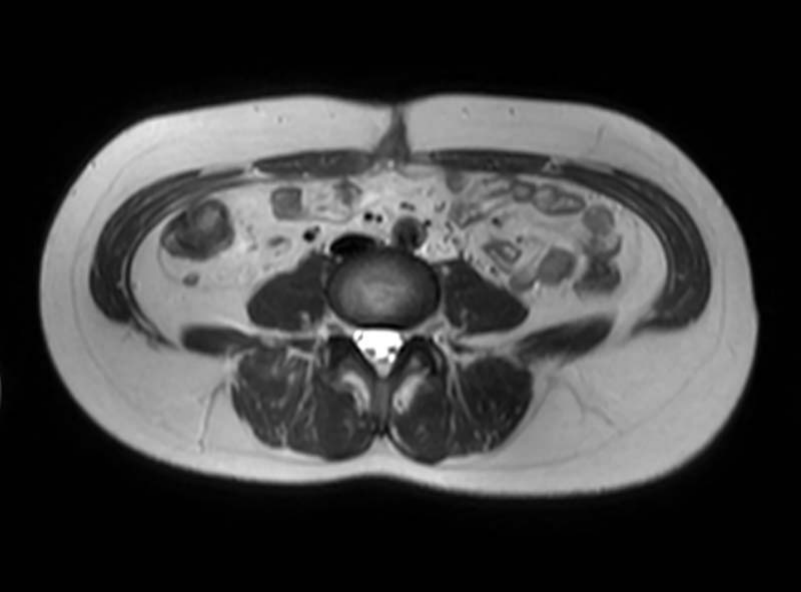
Postoperative MRI. Axial view.
